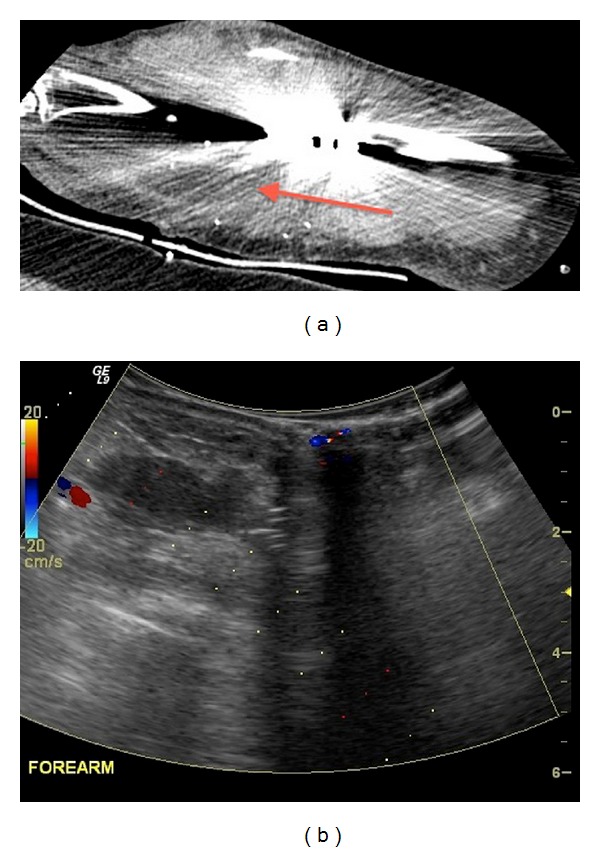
Postsurgical hematoma, questioned on CT and confirmed by ultrasound, as CT evaluation was significantly degraded by artifact from surgical hardware.
